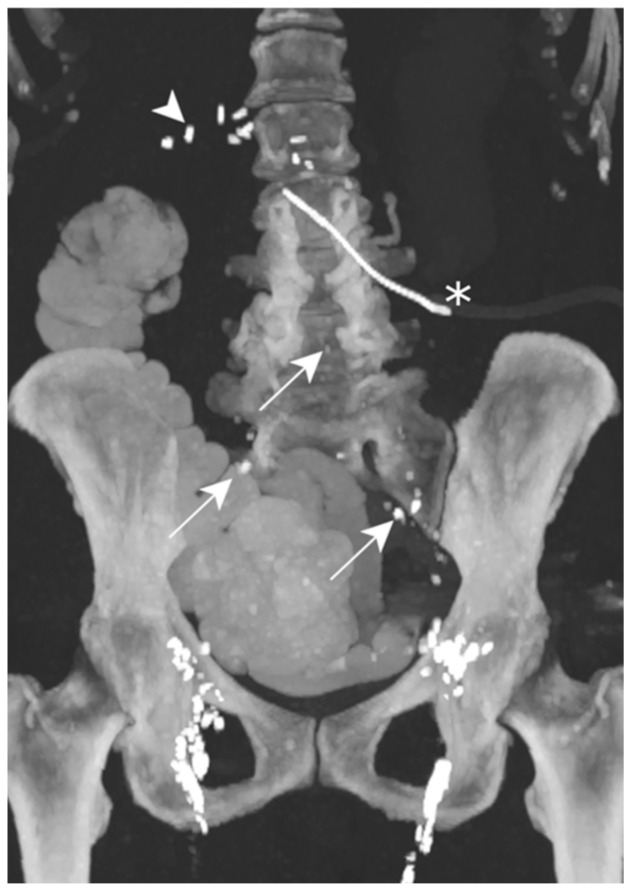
Patient E. The maximal-intensity projection of the CT scan reconstructed in the coronal plane after a transnodal Lipiodol injection, showing contrast agent in the external and common iliac as well as retroperitoneal (white arrows) lymphatic chains. No leakage of contrast agent is visualized. The presence of radiopaque surgical material is evident in the projection of the upper right quadrant, and is related to the cephalic duodenopancreatectomy (white arrowhead). The peritoneal drainage catheter (white asterisk) is identified.
